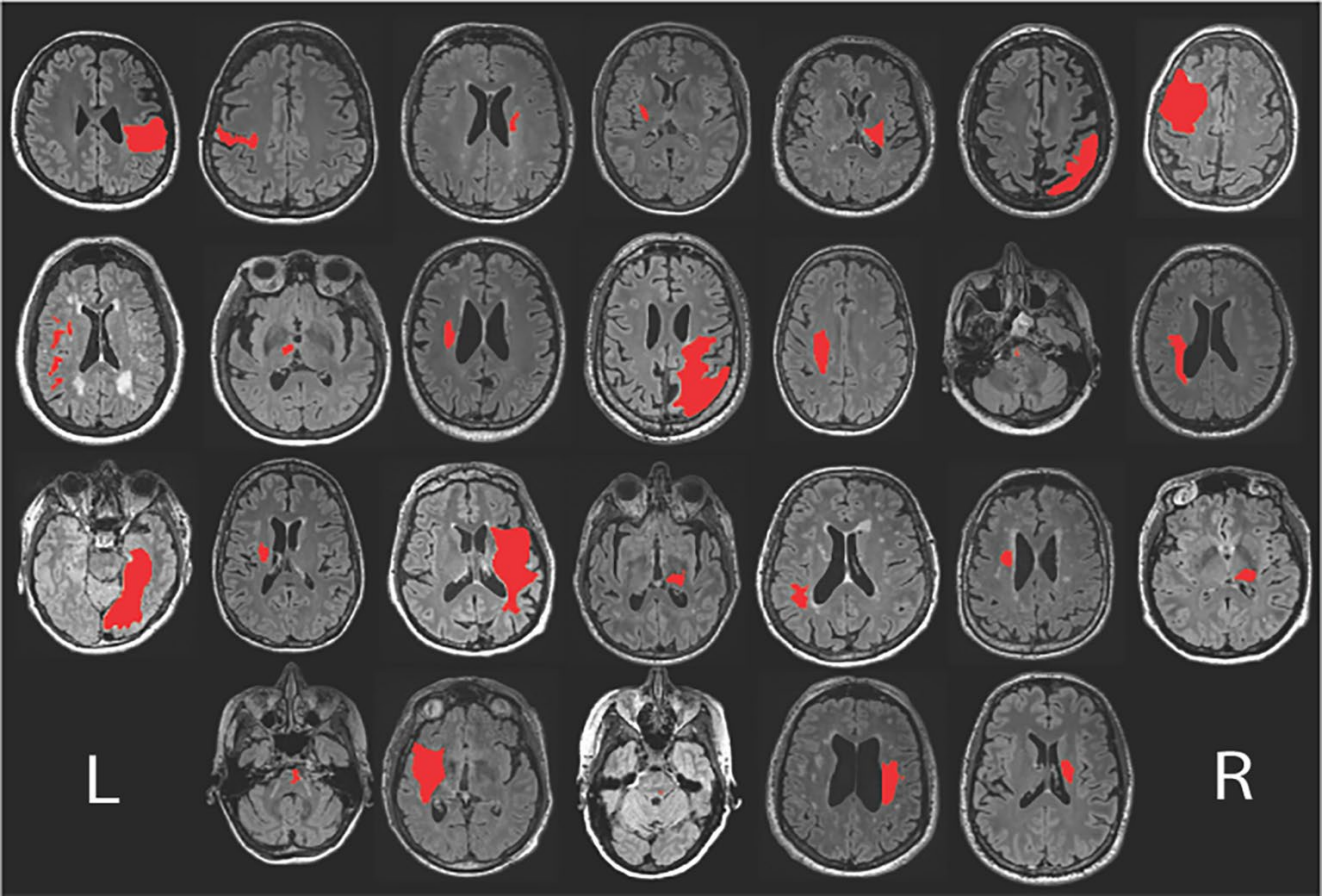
Individual MRI data showing level of greatest cross-sectional area of lesion. Lesion is shown in red.
